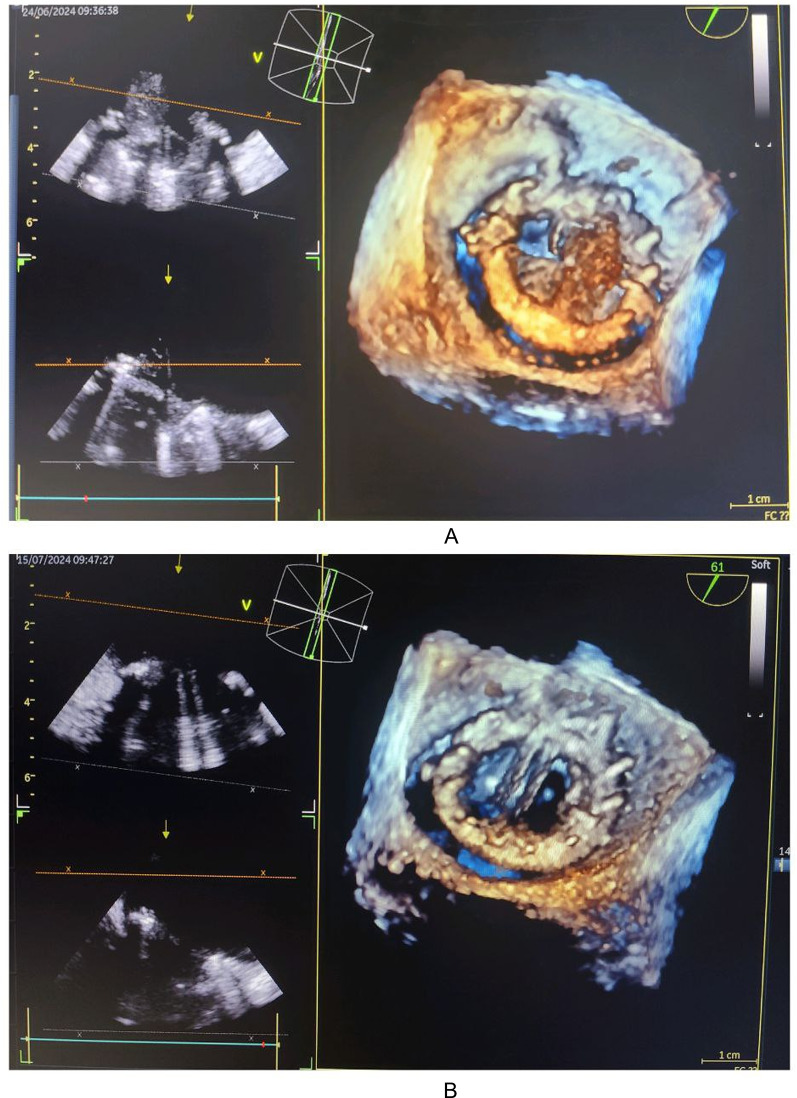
Trans-esophageal 3D echocardiography views at 45° (A) and 61° (B) before and after treatment, demonstrating significant regression of the vegetation and resolution of posterior leaflet obstruction
